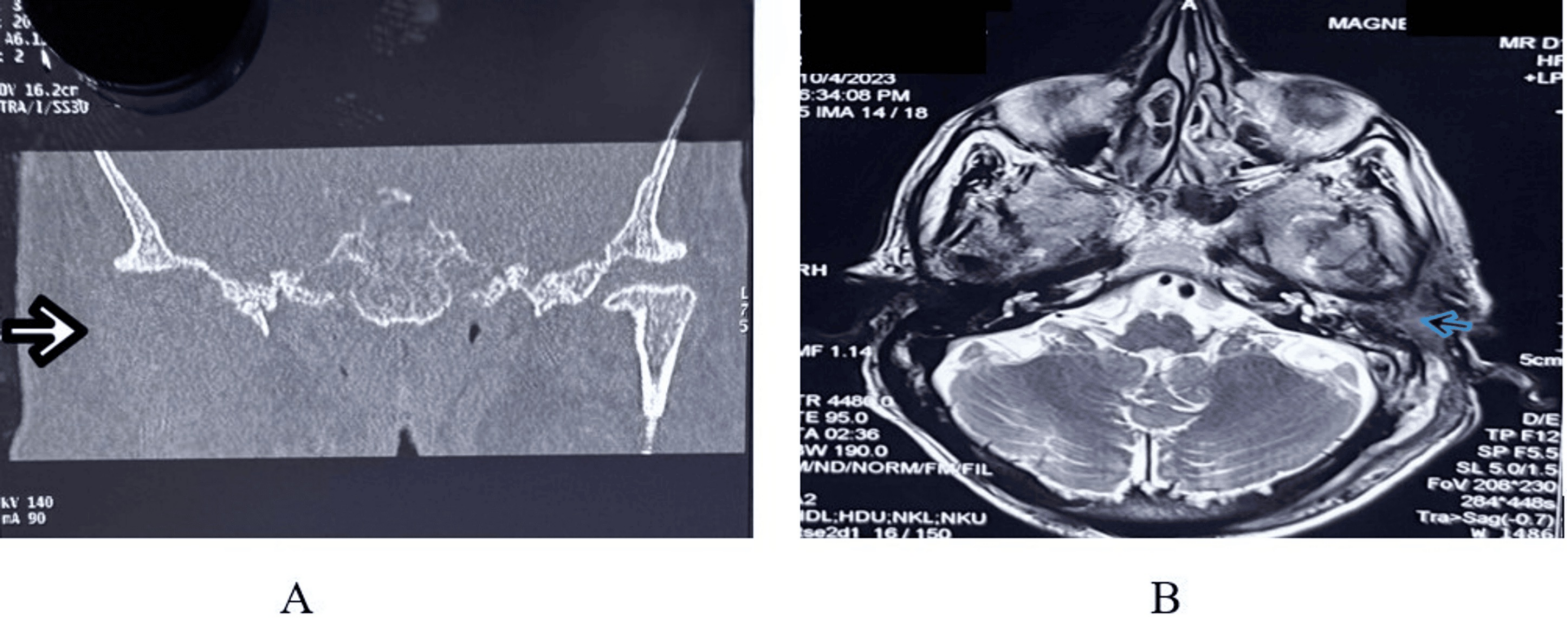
A: High-resolution computed tomography showing right TMJ septic arthritis. B: Magnetic resonance imaging of the brain A: High-resolution computed tomography showing right TMJ septic arthritis and anterior dislocation of the right TMJ. B: T2-weighted MRI showing hyperintense signal due to diffuse fluid accumulation filling the left middle ear cavity, mesotympanum, posterior epitympanum, and retrotympanic recess, with extension into the peri-ossicular region abutting the facial nerve canal. TMJ: temporomandibular joint
